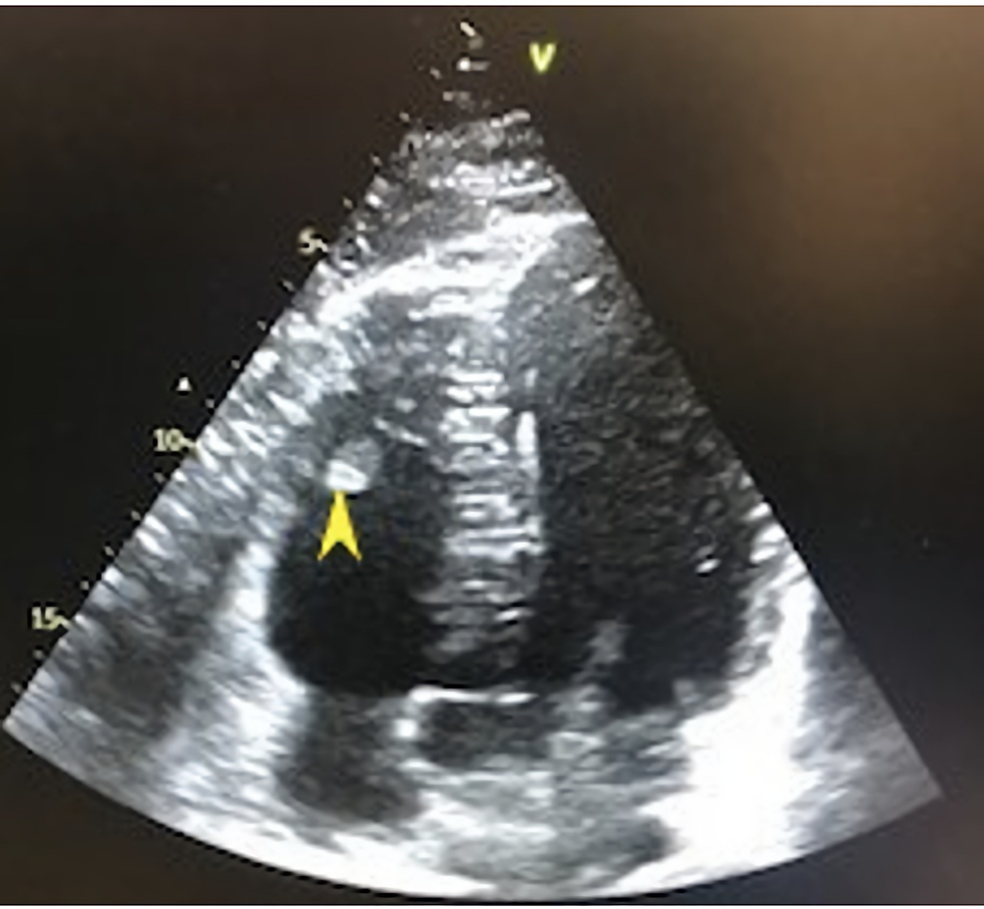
Echocardiogram showing clot in the right ventricle (yellow arrowhead)
